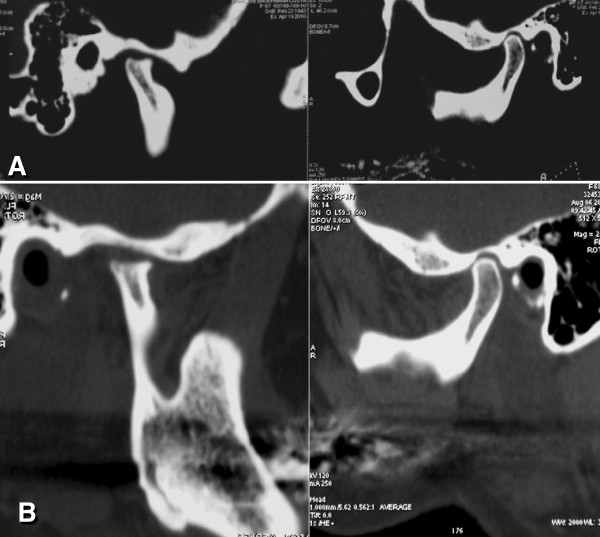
Pre- and postoperative CTs. (A) Pre-operative saggital-CT images (sections in closed-mouth position) suggested degenerative changes in right condyle. Left side showed no changes. (B) Post-operative sagittal CT: At post-operative period of 16 months, image showed no indication of progression of the degenerative changes in the right or left condyle.
